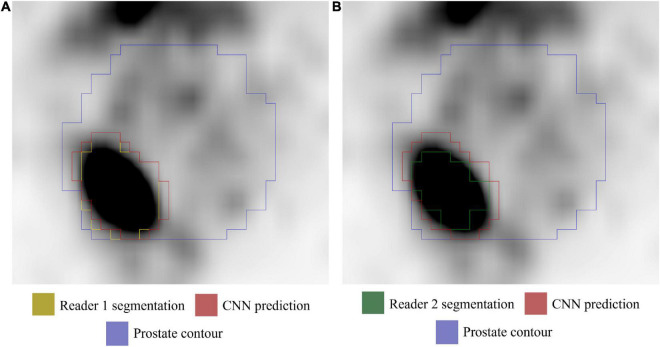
Axial ^68^Ga-PSMA PET image (image windowing SUVmin-max: 0–5). (A) GTV-Exp 1 lesion contour (yellow). (B) GTV-Exp 2 lesion contour (green). The GTV-CNN contour is shown in red and the prostate contour in purple.
